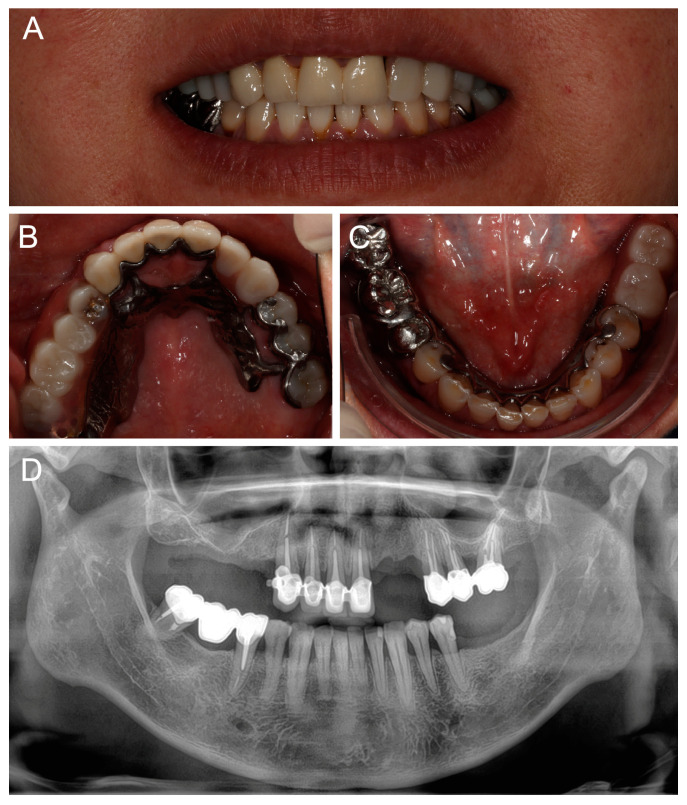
Follow-up at 2 years: (A) smile view; (B,C) occlusal views of maxillary and mandibular definitive prostheses; and (D) panoramic radiography.
